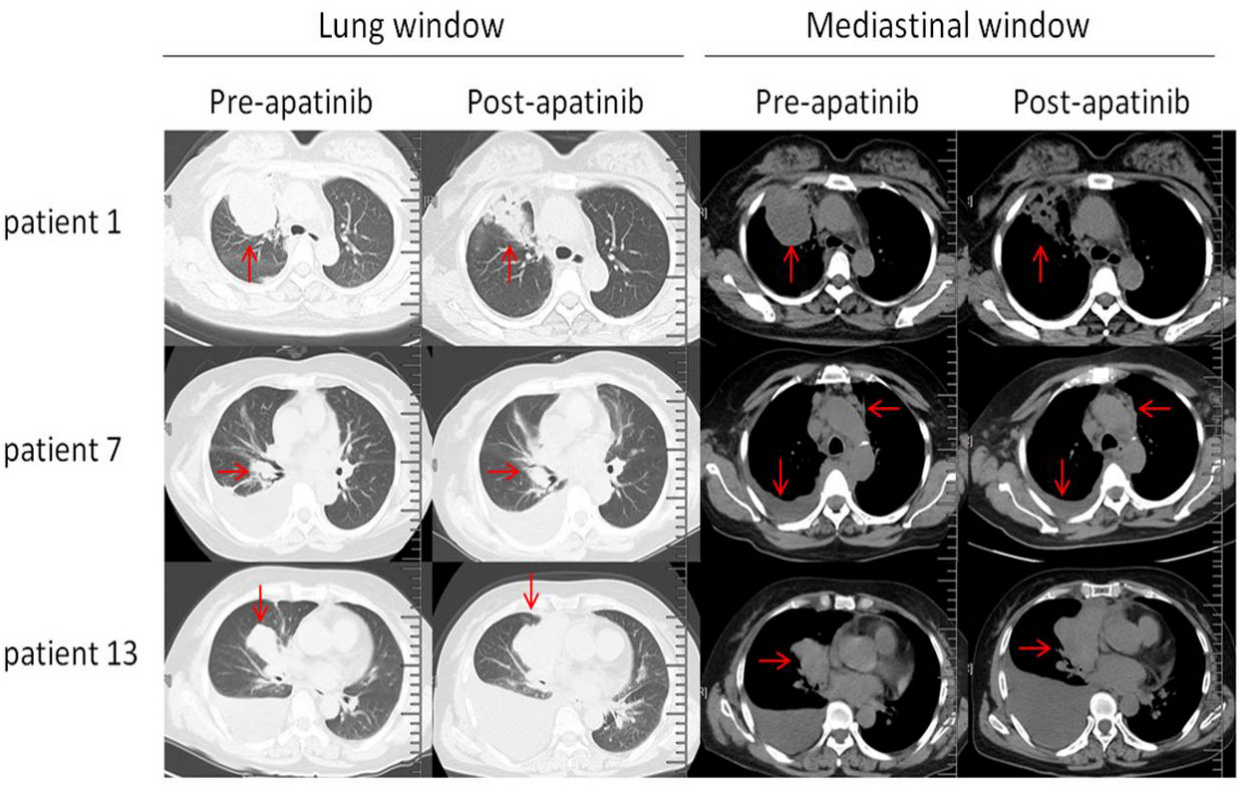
Representative CT images (lung windows and mediastinal windows) of three patients pre-apatinib and post-apatinib monotherapy CT images of patient 1 showed partial response of tumor in right upper lung (arrows). CT images of patient 7 showed stable disease response. Arrows showed the similarity of tumor in right middle lobe lung, enlarged lymph nodes and pleural effusion. CT images of patient 13 showed progressive disease response of tumor in right middle lobe lung and pleural effusion (arrows).
